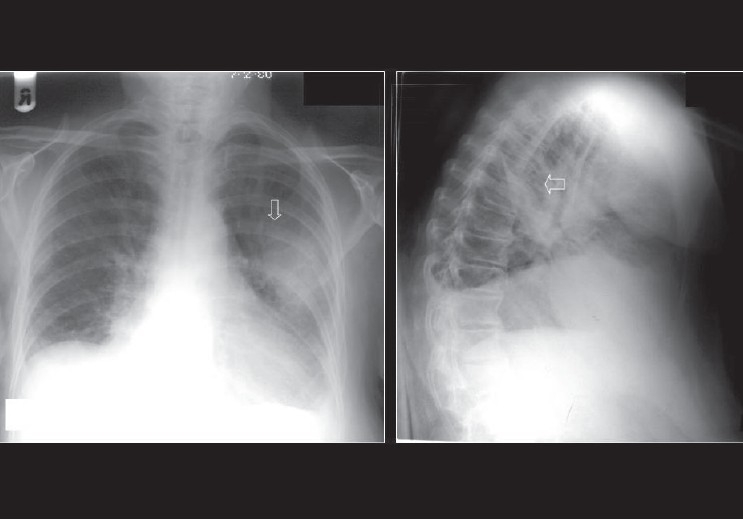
Encysted pleural effusion seen en face as an oval opacity; its margin is partially well defined and partially ill defined (AP radiograph). On the lateral radiograph, the effusion appears as a homogenous density with biconvex edges
